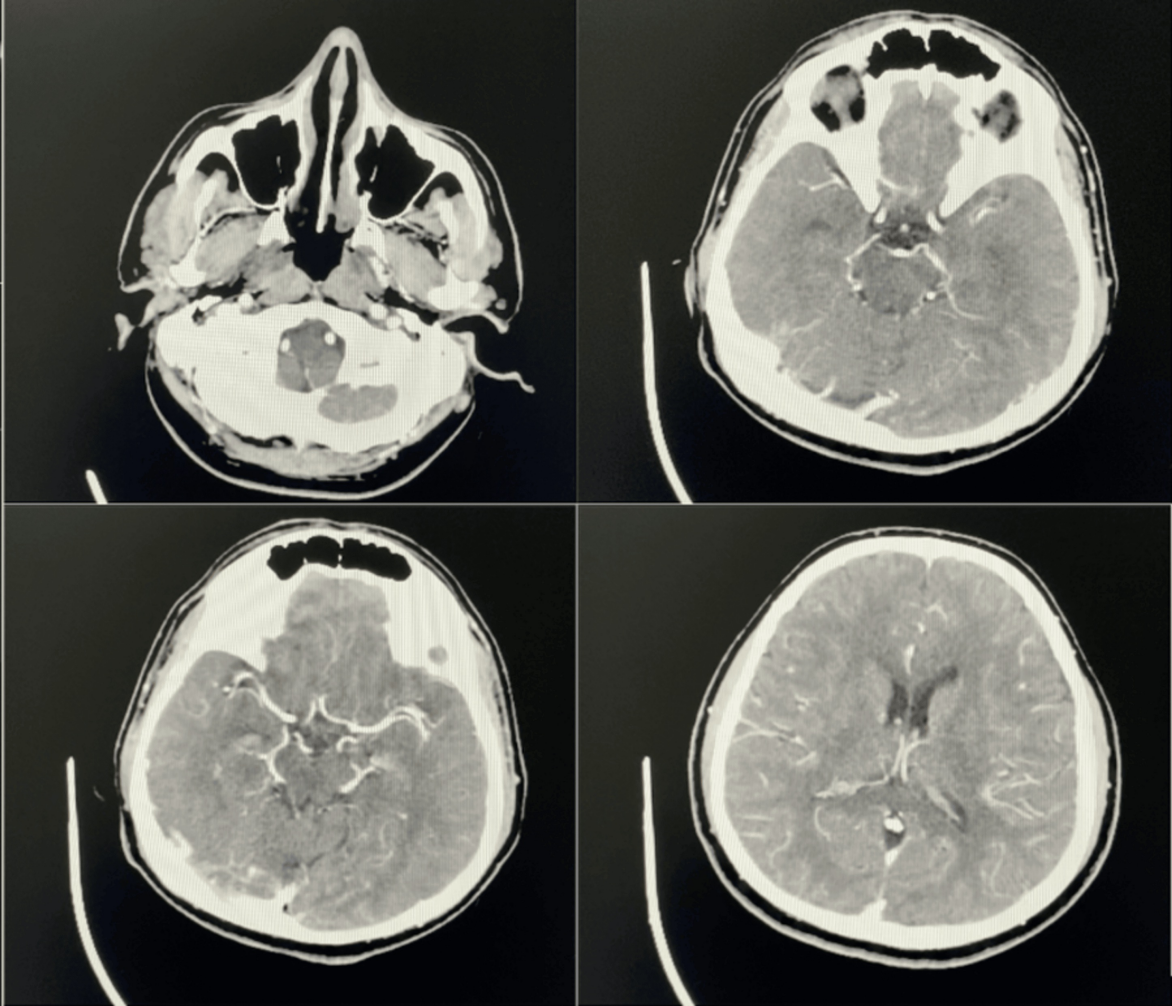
Computed tomography angiography showed that no significant stenosis was detected in major branches
